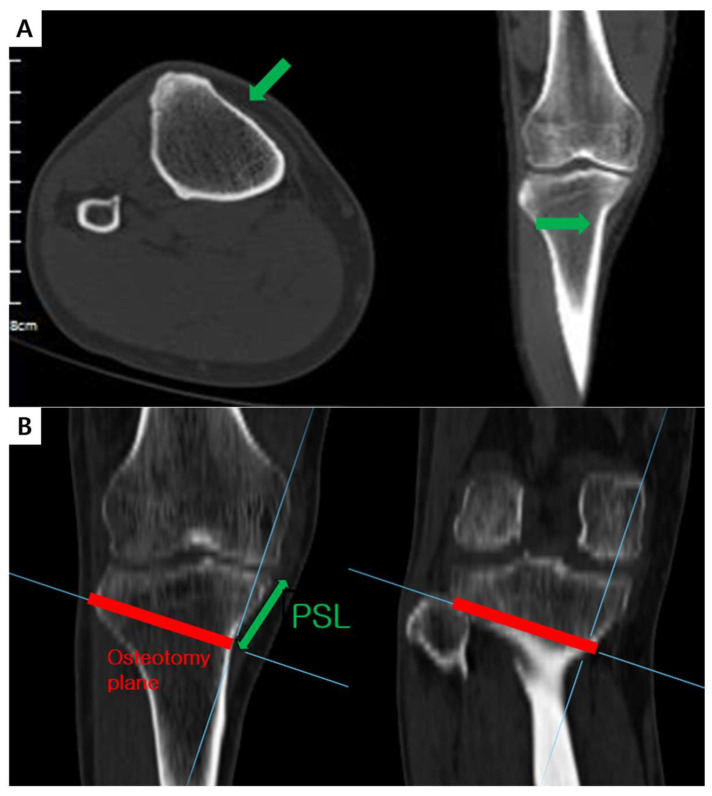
(A) Identification of the starting point of the osteotomy plane just above pes insertion on the 3D computed tomography scan. (B) Assuming the osteotomy plane from the osteotomy starting point to the proximal tibiofibular joint. After that, the proximal segment length (PSL) was measured.
